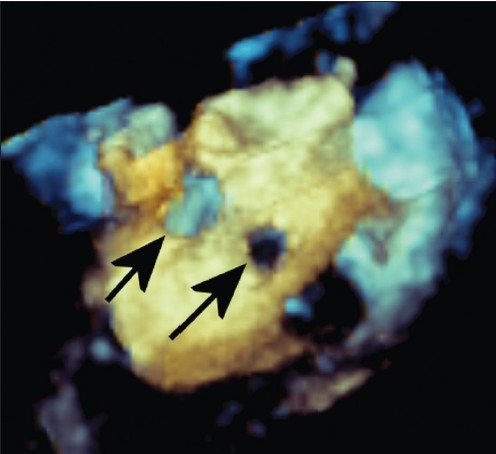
Matrix-array three-dimensional transesophageal echocardiogram obtained at the level of the mid esophagus, directly behind the anatomic left atrium. One larger and one smaller baffle leak are demonstrated (arrows)
